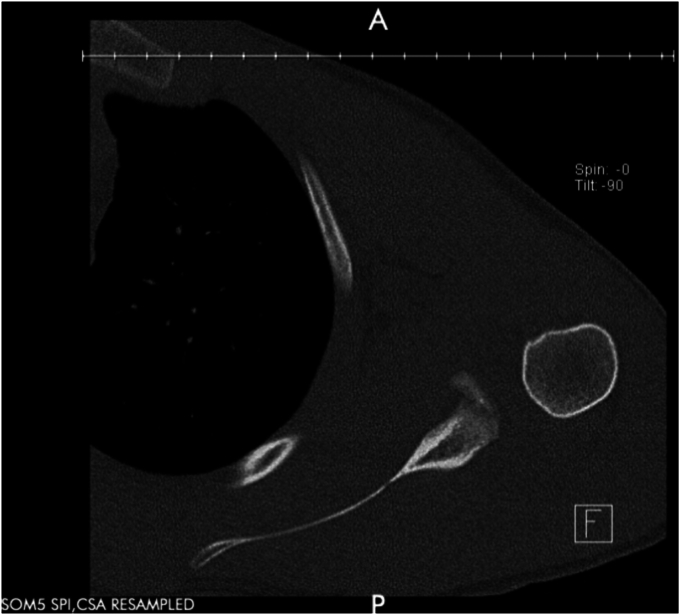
Axial CT image taken after anterior dislocation revealing bony Bankart lesion.
